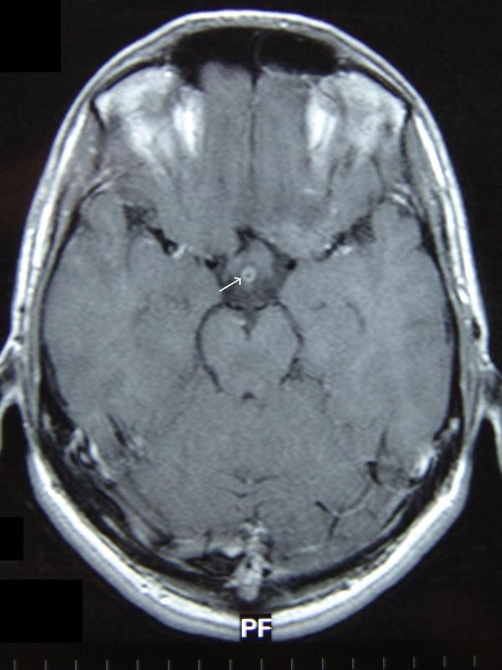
Magnetic resonance image demonstrating cerebral vasculitis.
